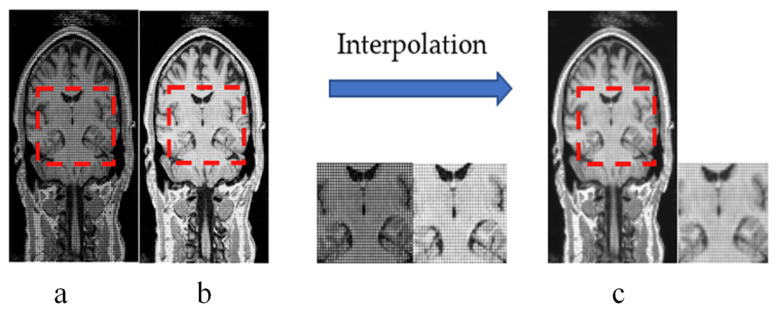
(a,b) Adjacent slices which are from with different brightness; Slices belong to the newly reconstructed MRI in the first reconstruction. (c) New slice after interpolation repair. Noise and grid lines still exist from traditional interpolation. Interpolation only solves the problem of brightness and recovers only a few details.
